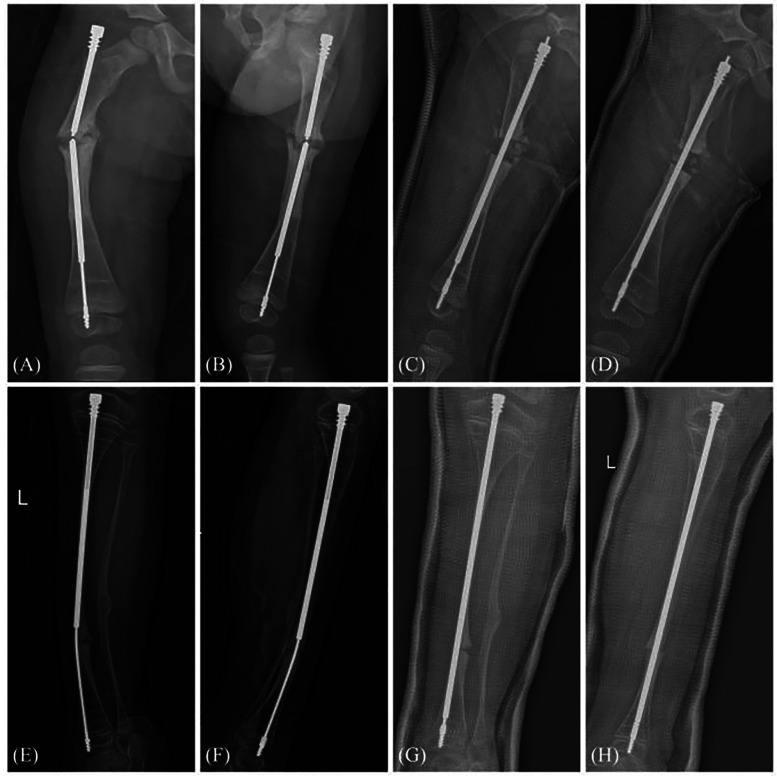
Radiographs showing complications at 6 months postoperatively in the right femur of a 2-year-old patient. (A,B) Anteroposterior and lateral views demonstrating fracture of the right femoral FD nail. (C,D) Immediate postoperative anteroposterior and lateral views following revision surgery for right femoral FD nail fracture. X-ray showing complications at 39 months postoperatively in an 8-year-old patient. (E,F) Anteroposterior and lateral views showing bending of the left tibial FD nail. (G,H) Anteroposterior and lateral views immediately after revision surgery for bending of the left tibial FD nail. Both patients received replacement FD nails.
